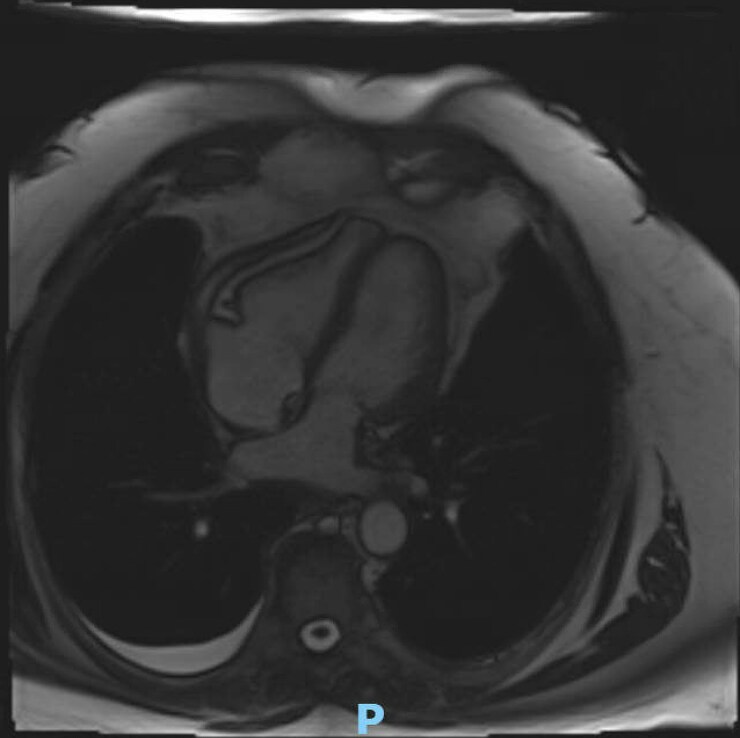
Cardiac magnetic resonance imaging showing pericardial thickening with septal flattening.
